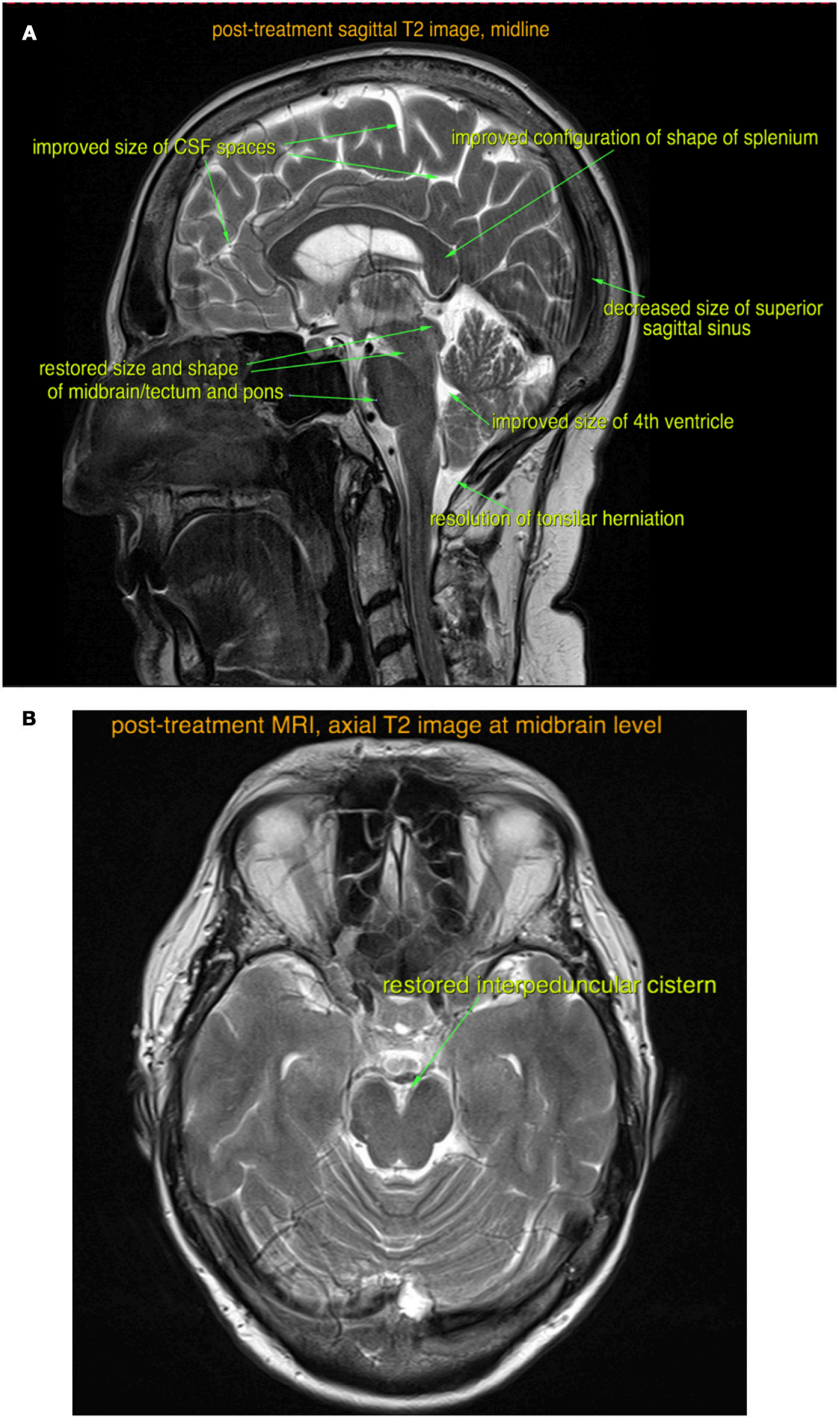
Post-treatment sagittal (A) and axial (B) T2-weighted MRI images show almost complete resolution of the findings of intracranial hypotension.
